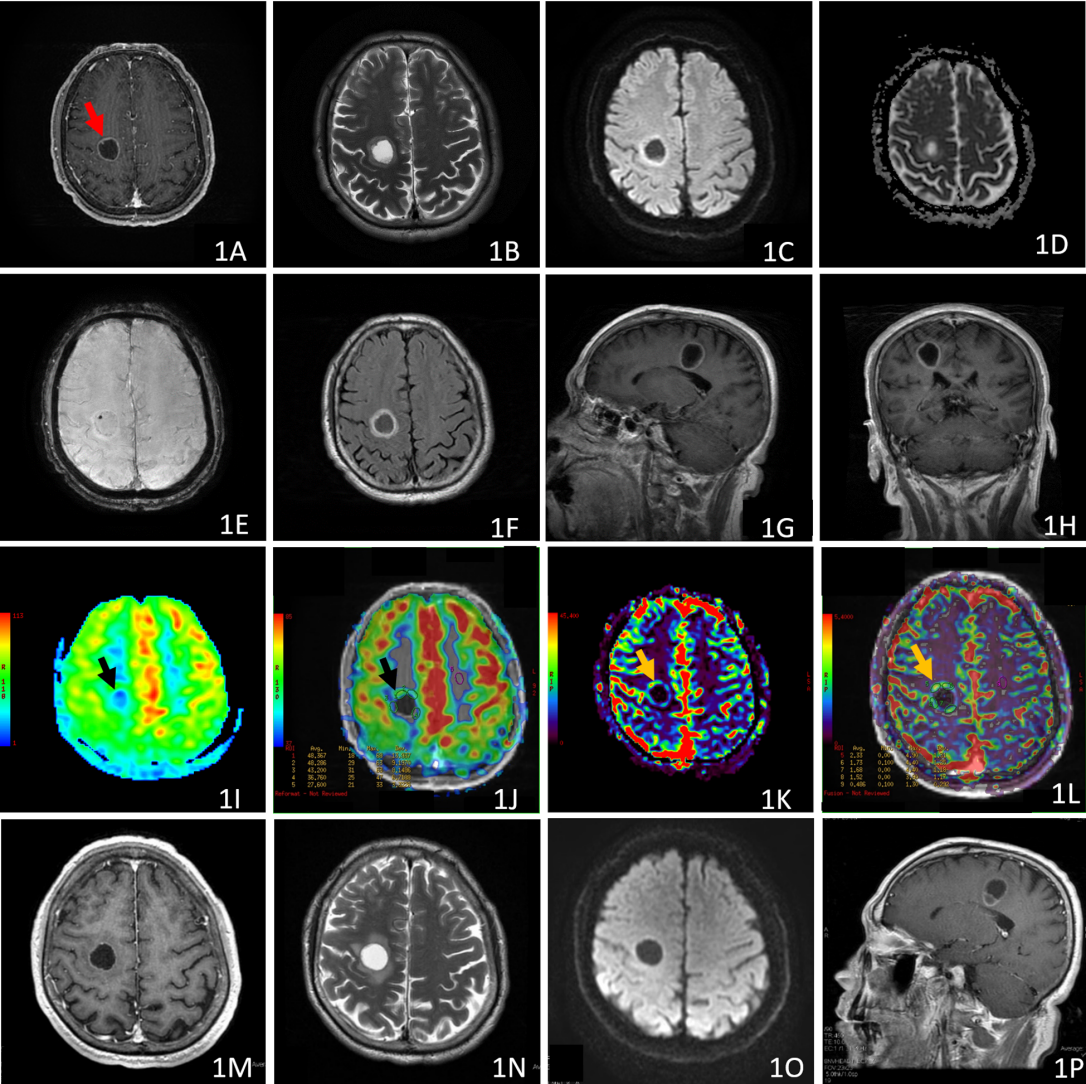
Case 1, axial post-contrast 3D T1-weighted (A), T2WI (B), DWI (C), ADC (D), SWI (E), T2-FLAIR (F), post-contrast sagittal T1-FLAIR (G), and post-contrast coronal T1-FLAIR (H) of the pre-treatment MRI revealed a ring-enhancing mass without peritumoral edema in right frontal lobe. The enhancement of the rim was heterogeneous, with an enhancing nodule of the inner wall [red arrow, (A)]. The ASL-CBF maps [black arrow, (I, J)] and DSC-PWI-CBV maps [yellow arrow, (K, L)] showed increased perfusion of the anterior enhancing rim. Post-contrast 3D T1-weighted (M), T2WI (N), DWI (O), and post-contrast sagittal T1-FLAIR (P), of follow-up MRI which was scanned three months after immunotherapy and radiation treatment,showed decreased enhancement of the mass with slight edema. The mass volume did not change appreciably.
